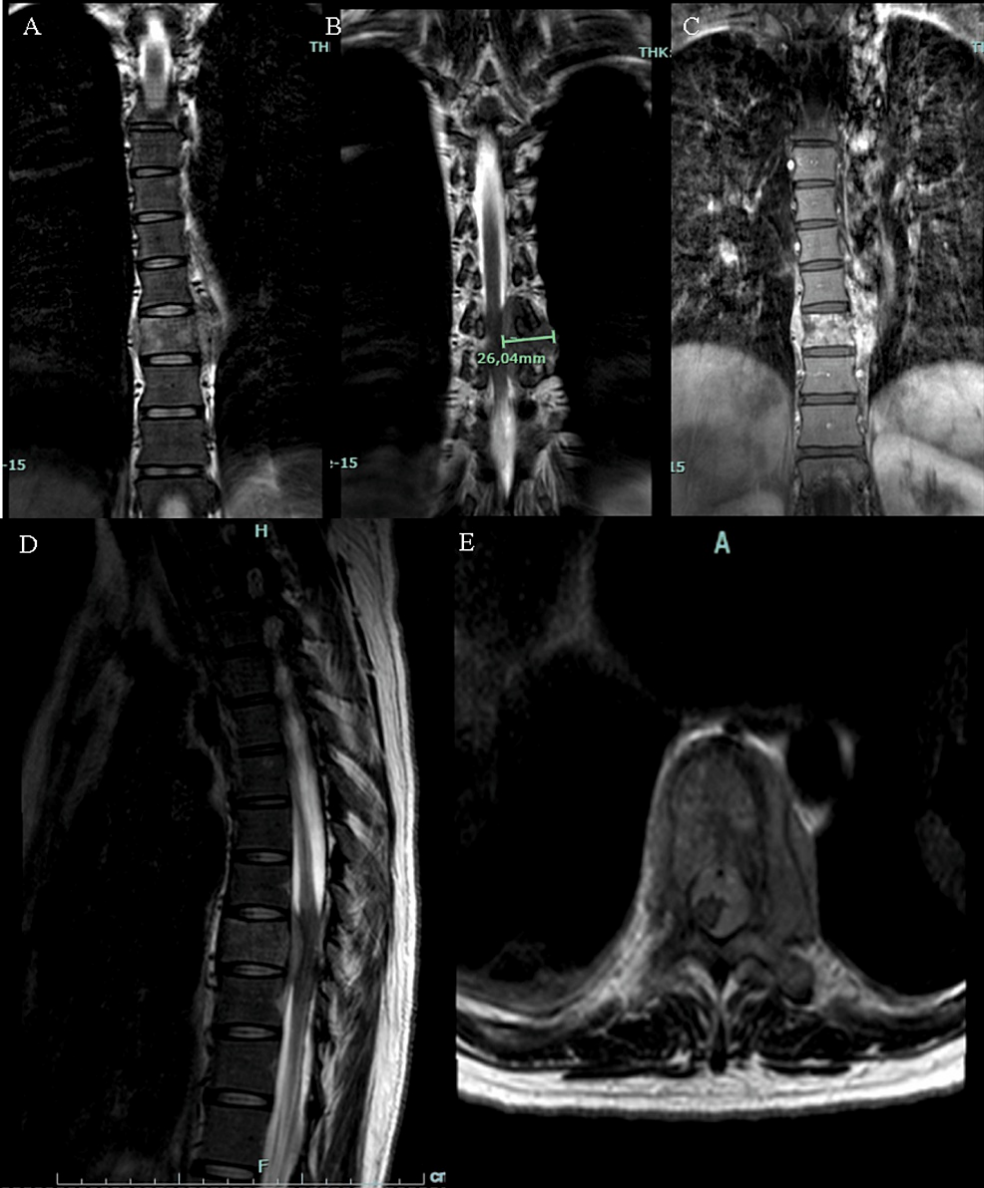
MRI of the thoracic and lumbar spine (A, B, C). A paravertebral lesion is observed at the level of the T9 thoracic vertebra with evidence of extension toward the epidural space and spinal cord compression predominantly on the left side. 1C shows a hyperintensity of the lesion in the T1W sequence. 1D shows a sagittal section showing a pathological fracture at the level of the T9 vertebral body with evidence of spinal cord compromise at that level. 1D shows an axial section at the level of the T9 vertebra. T1W: T1 weighted
